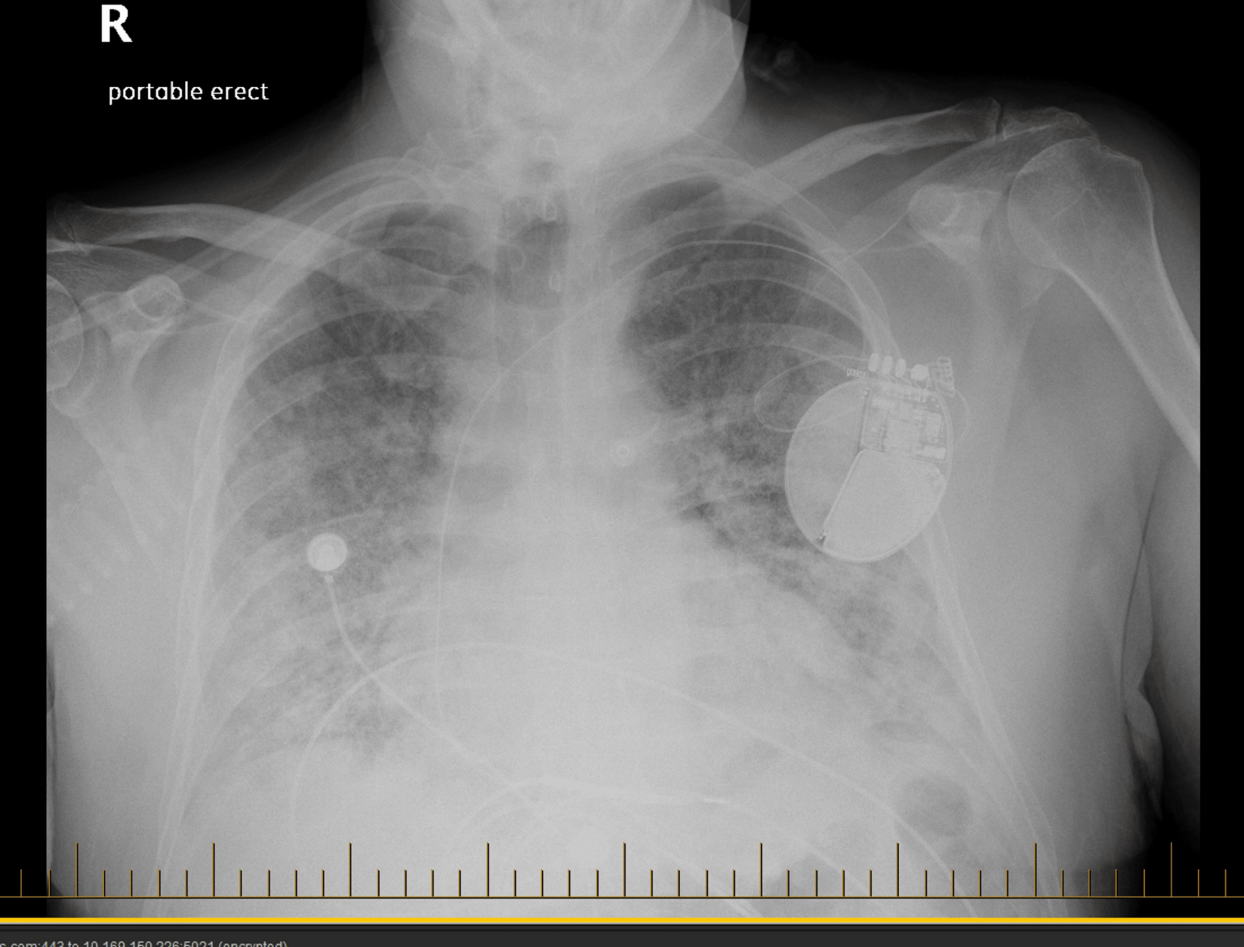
Portable erect chest X-ray demonstrating diffuse patchy opacities bilaterally, which appear unchanged from prior imaging No evidence of pleural effusion or pneumothorax is noted. The left chest wall pacemaker lead tips remain in a stable position.
